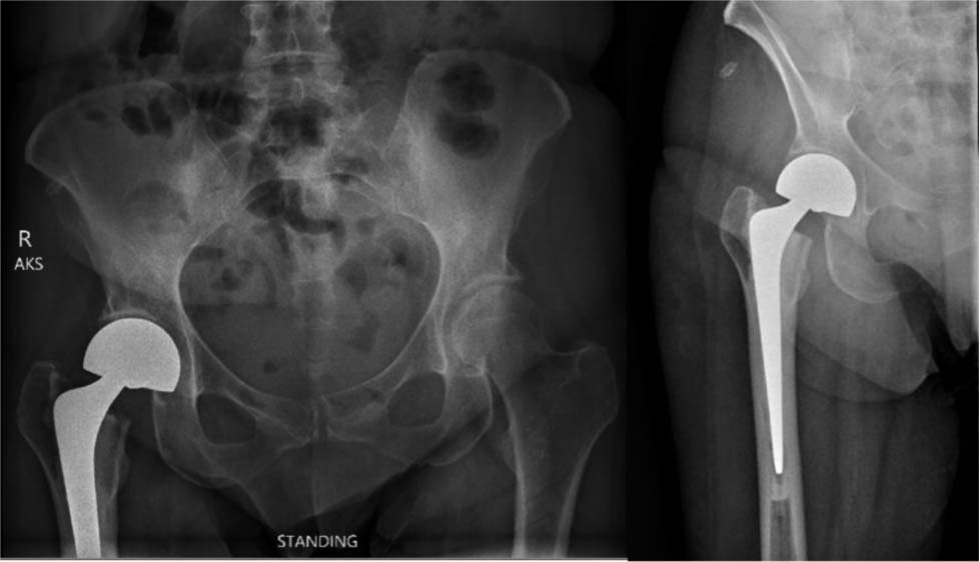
Postoperative anterior-posterior pelvis (left) and hip (right) radiograph demonstrating a cemented total hip arthroplasty with complete filling of the medullary canal.
